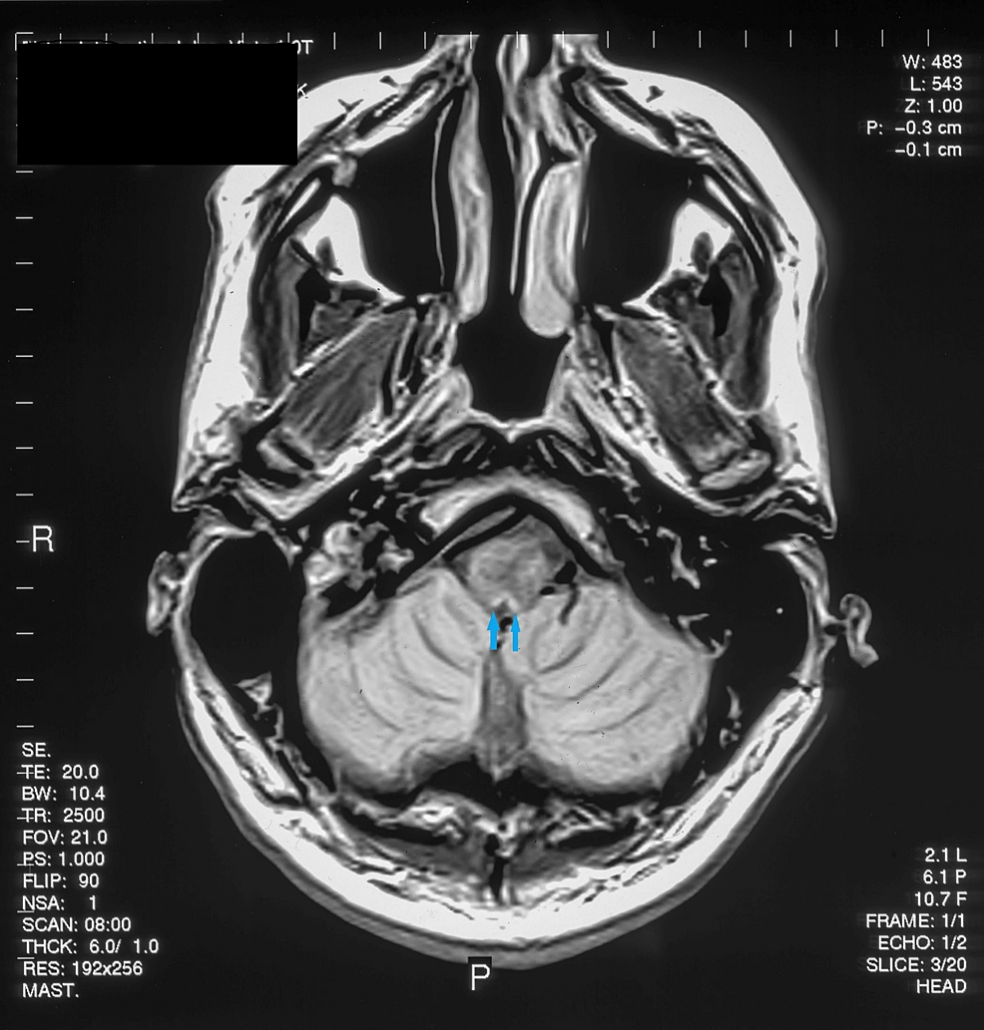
1T axial MRI T2-weighted image showing enhancement of area postrema bilaterally (blue arrows)
